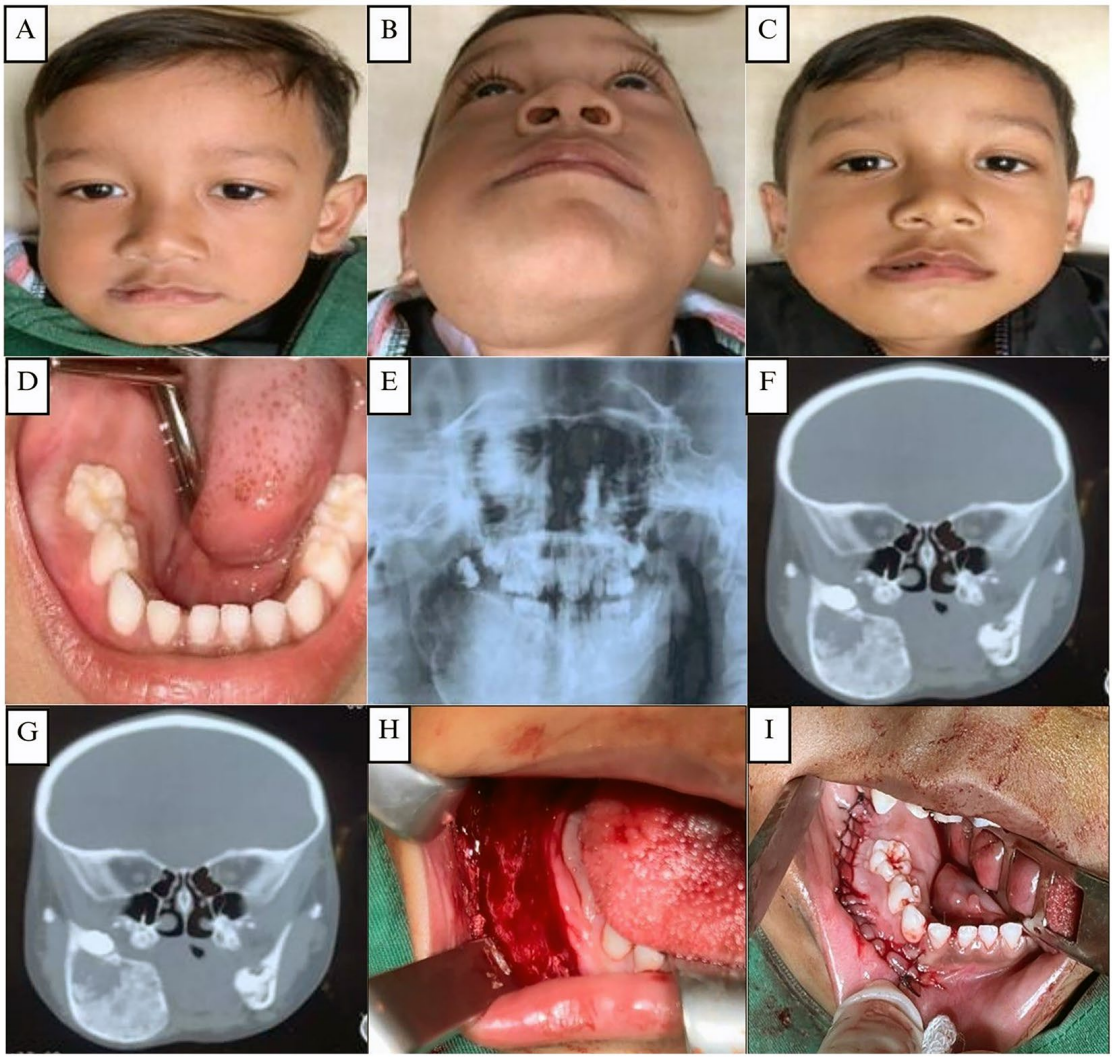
(A and B) The second case, a 4-year-old male child patient with swelling in the right mandibular area. (C) Clinical appearance 2 weeks post-operative. (D) Pre-operative intraoral clinical appearance (E). OPG showing a radiolucent lesion in the right mandibular area with an amorphous shape with multiple unerupted permanent teeth. (FG) CT scan showing a hard tissue mass in the right mandible area with cystic lesions, expansile lesions with a size of 37.3 × 45.7 mm, oval shape with firm boundaries. (H–I) Right mandibular intraoral area: surgical exposure, bone reshaping, and layers of closure.
